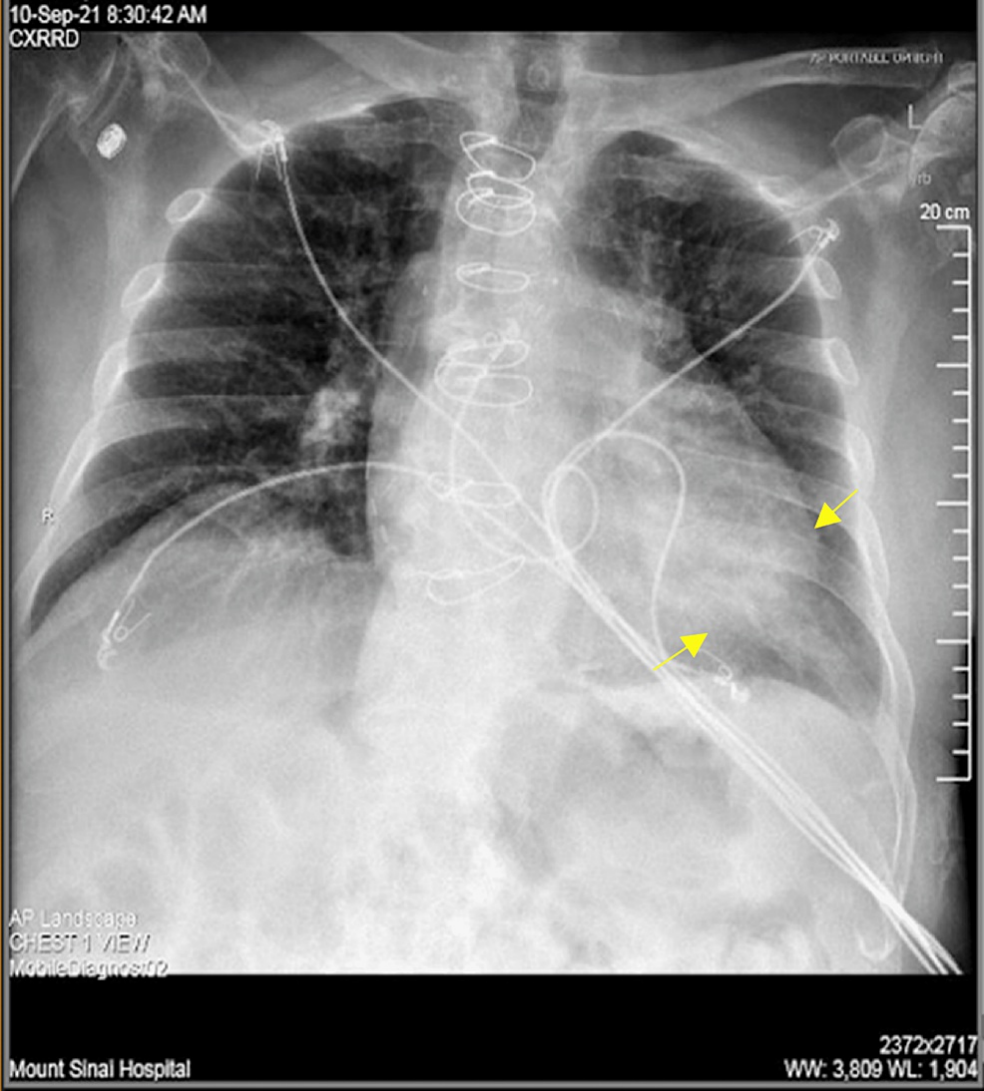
Chest radiograph When compared with the previous study, there is mildly improved aeration at the left lung base (yellow arrows)
